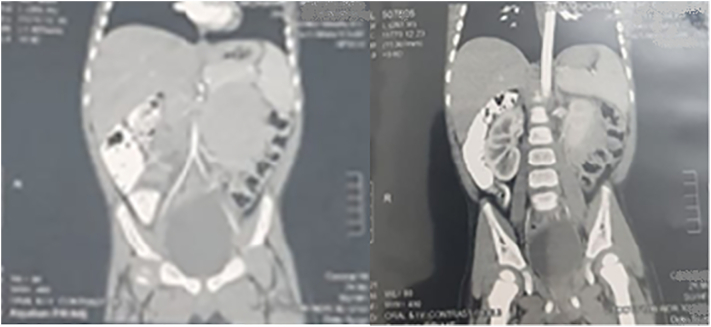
A cluster of small intestine loops were seen at the left anterior para-renal space on postcontrast CT abdomen and pelvis sagittal plane.
